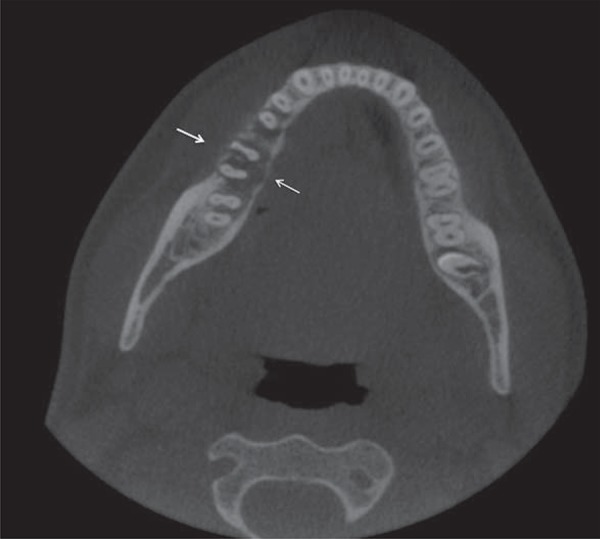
Axial plane cone beam computed tomography showing a thinning of the buccal and lingual cortical plates (arrows).
